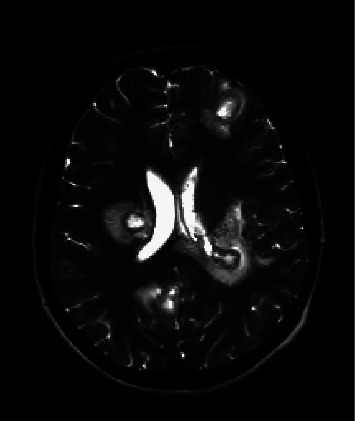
Brain magnetic resonance imaging demonstrating ring-enhancing lesions throughout the brain parenchyma and cerebellum consistent with abscesses on admission.
